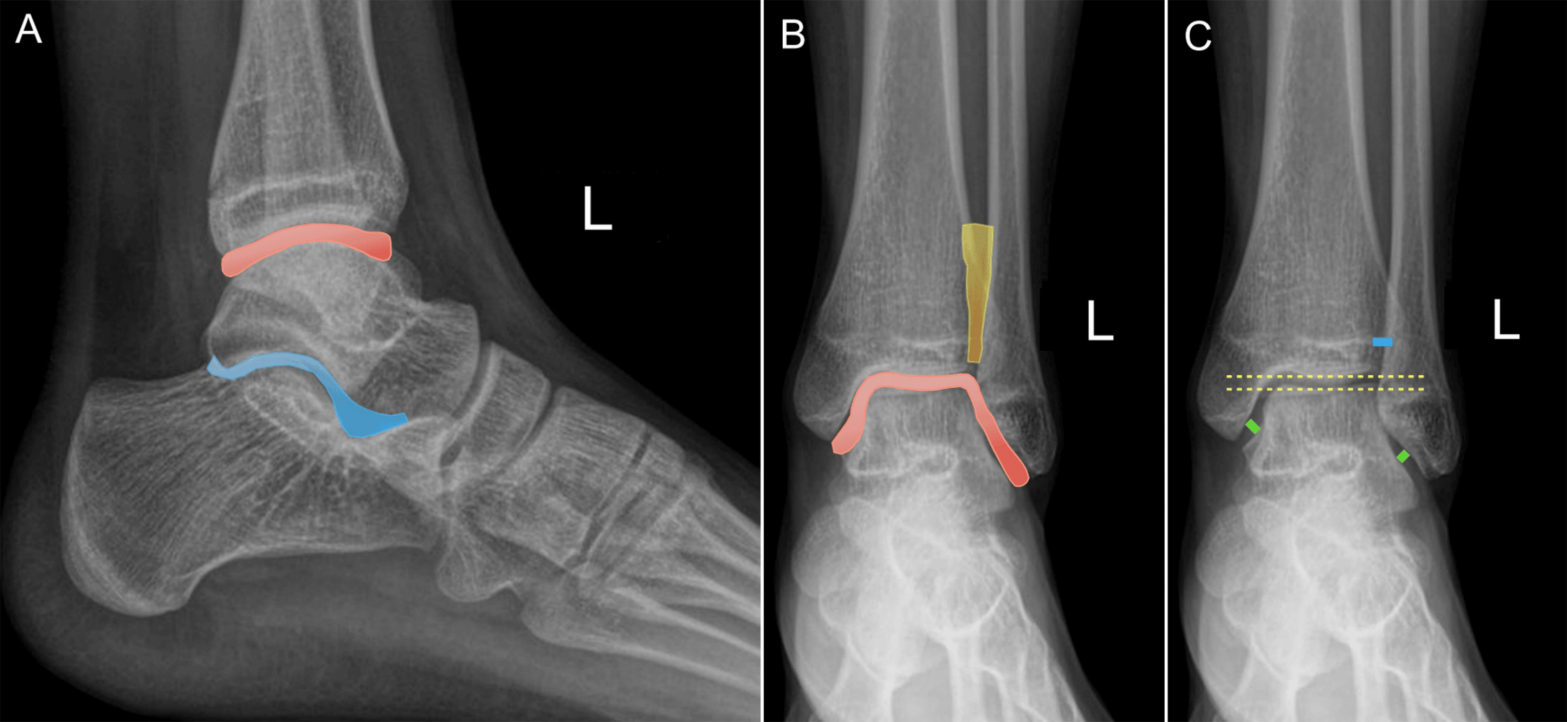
Normal ankle radiographs in a 16-year-old female. Anteroposterior and lateral radiographs demonstrate (A, B) the tibiotalar joint (red highlight), subtalar joint (blue), and syndesmotic joint (yellow); (C) normal tibio-talar parallelism (yellow dashed line), uniform joint space (green lines), and tibiofibular space (blue line). Images belong to the authors; patient consent was obtained for publication.
